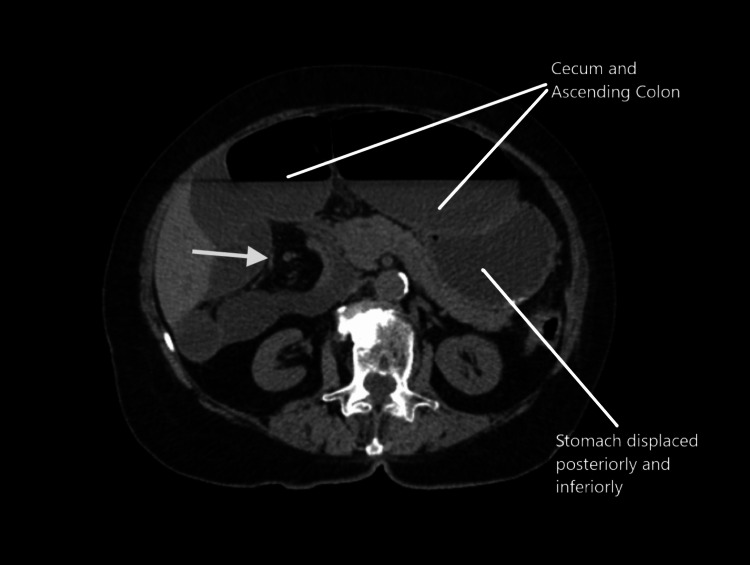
CT abdomen and pelvis without contrast. Whirl sign displaying cecal volvulus (arrowhead).
